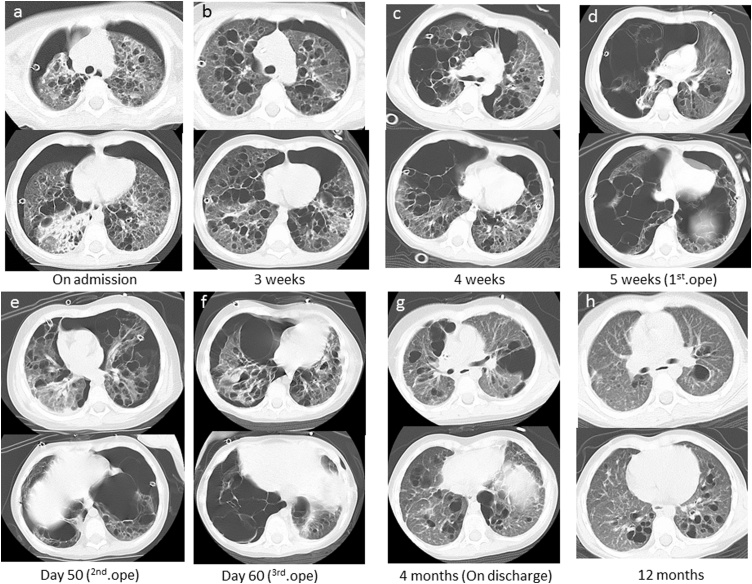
Series of patient’s chest CT.
